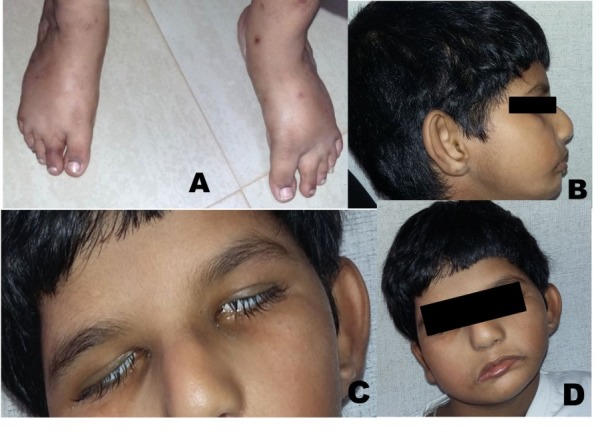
Wrist radiographs showed no abnormality (Figure 5, A). A lateral cephalogram showed beaten metal appearance of skull and multiple surgical plates and screws (Figure 5, B). A multidisciplinary approach was established for the treatment of the patient. He is under regular follow-up.
